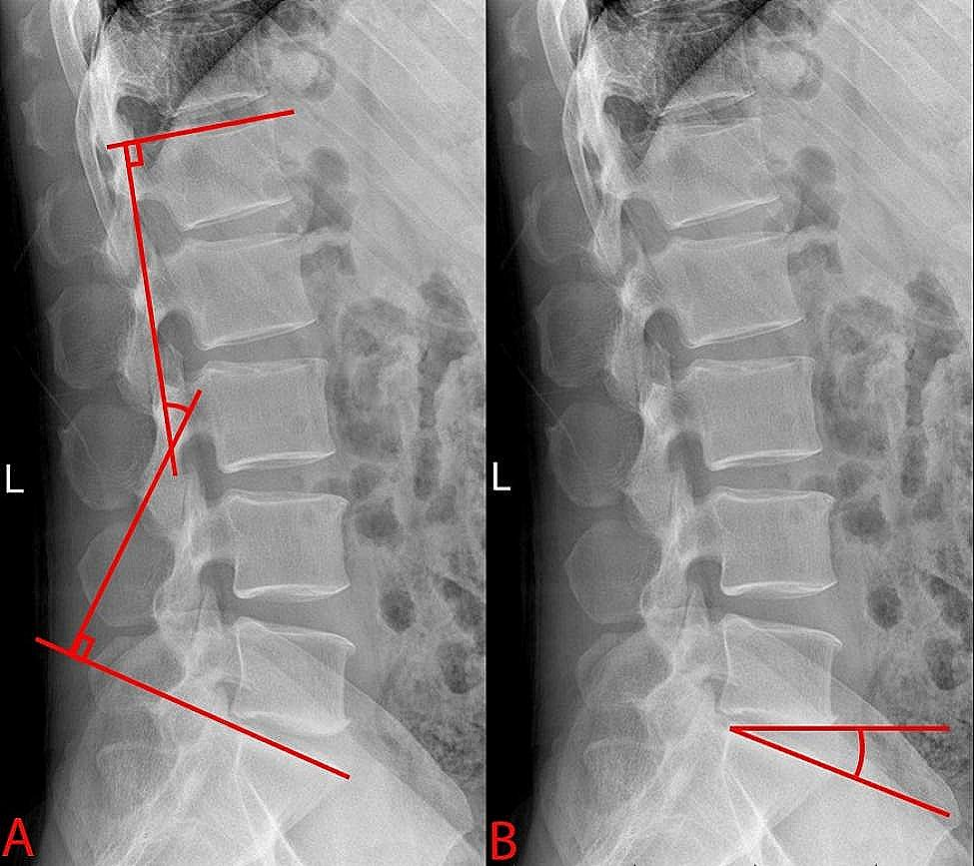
The measurement method of lumbar lordosis angle and sacral slop angle. *Note*. Standing radiograph shows lumbar lordosis angle (A) and sacral slope angle (B)
